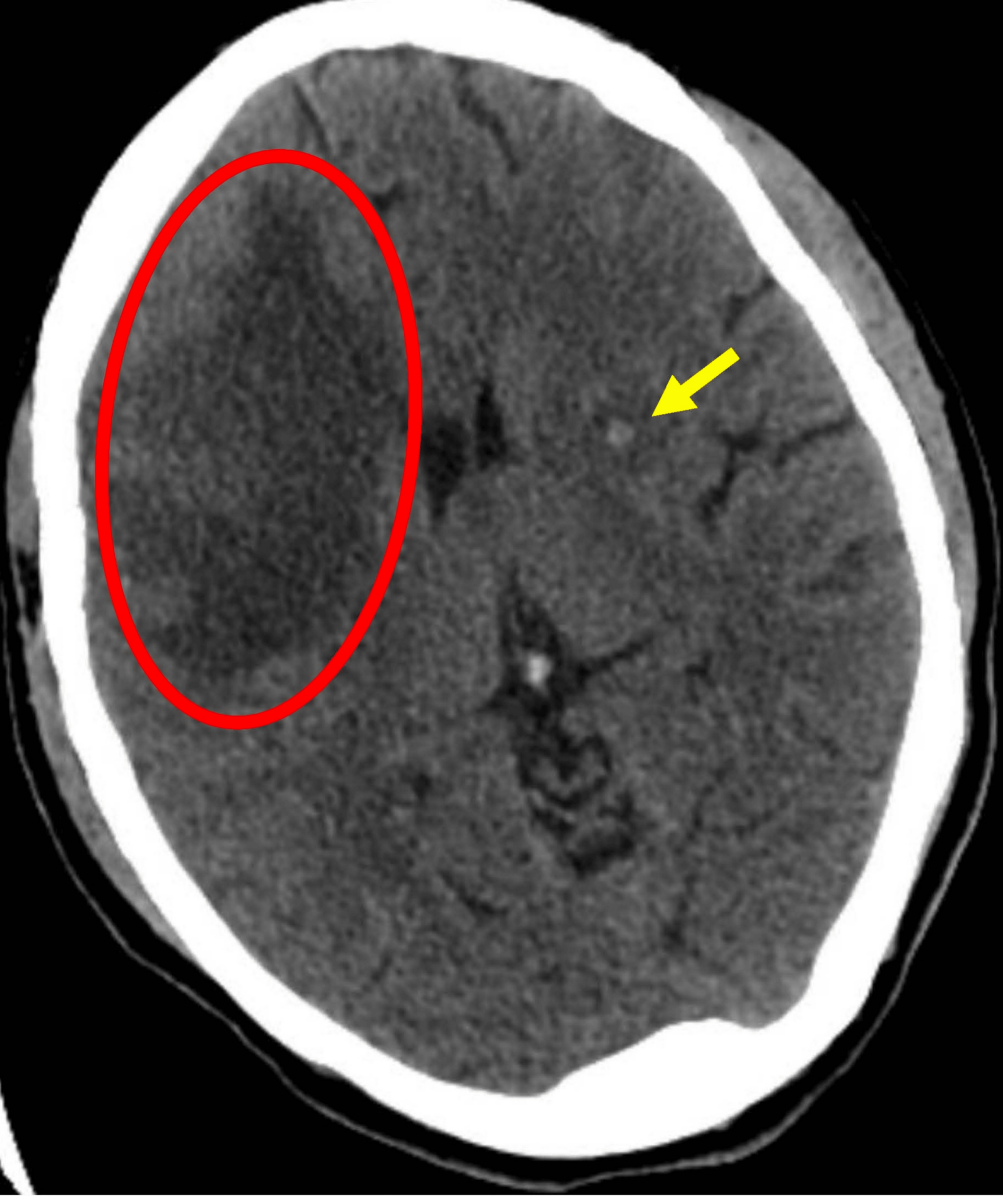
Initial head CT The yellow arrow indicates a 4 mm lesion in the left basal ganglia. The red circle represents a region of edema in the right frontal lobe.
